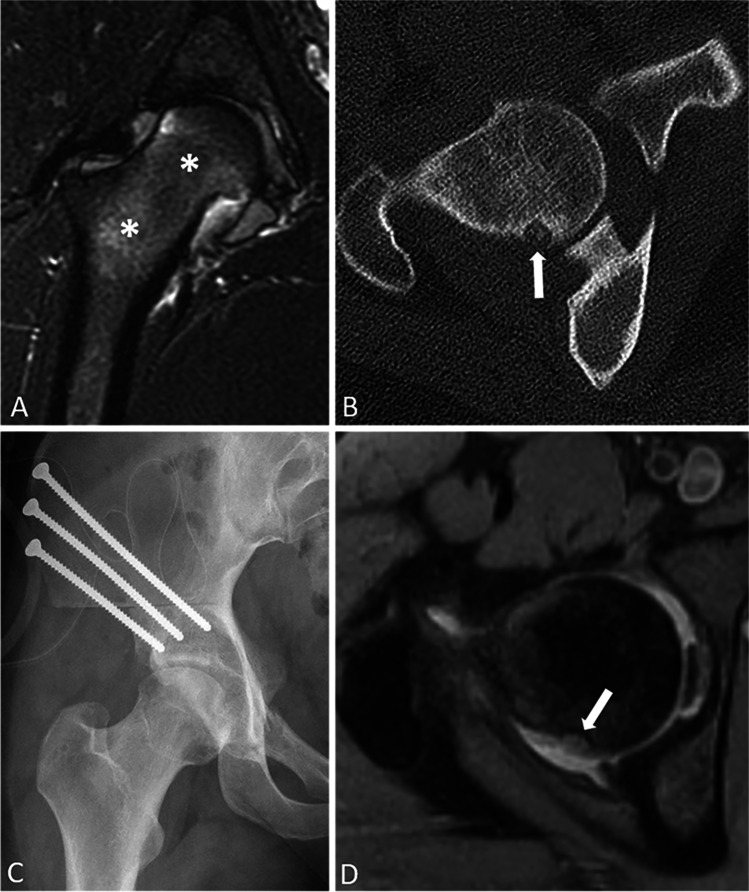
Coronal STIR MR image of the right hip (A) of an 18-year-old female shows a joint effusion, synovitis, and bone marrow edema in the head and neck of the femur (asterisks), along with a labral tear and cam deformity (not shown). Patient was treated with hip arthroscopy and head-neck osteoplasty with no appreciable change in pain. Axial CT (B) obtained for pre-operative planning for subsequent periacetabular osteotomy (C) shows an OO near the head-neck junction of the femur posteriorly (arrow). In retrospect, the OO was visible on the original axial proton density MRI (D), but easy to miss if not entertaining the diagnosis on the differential. Patient reported pain at night relieved with NSAIDs, which was only relieved following RF ablation of the OO
